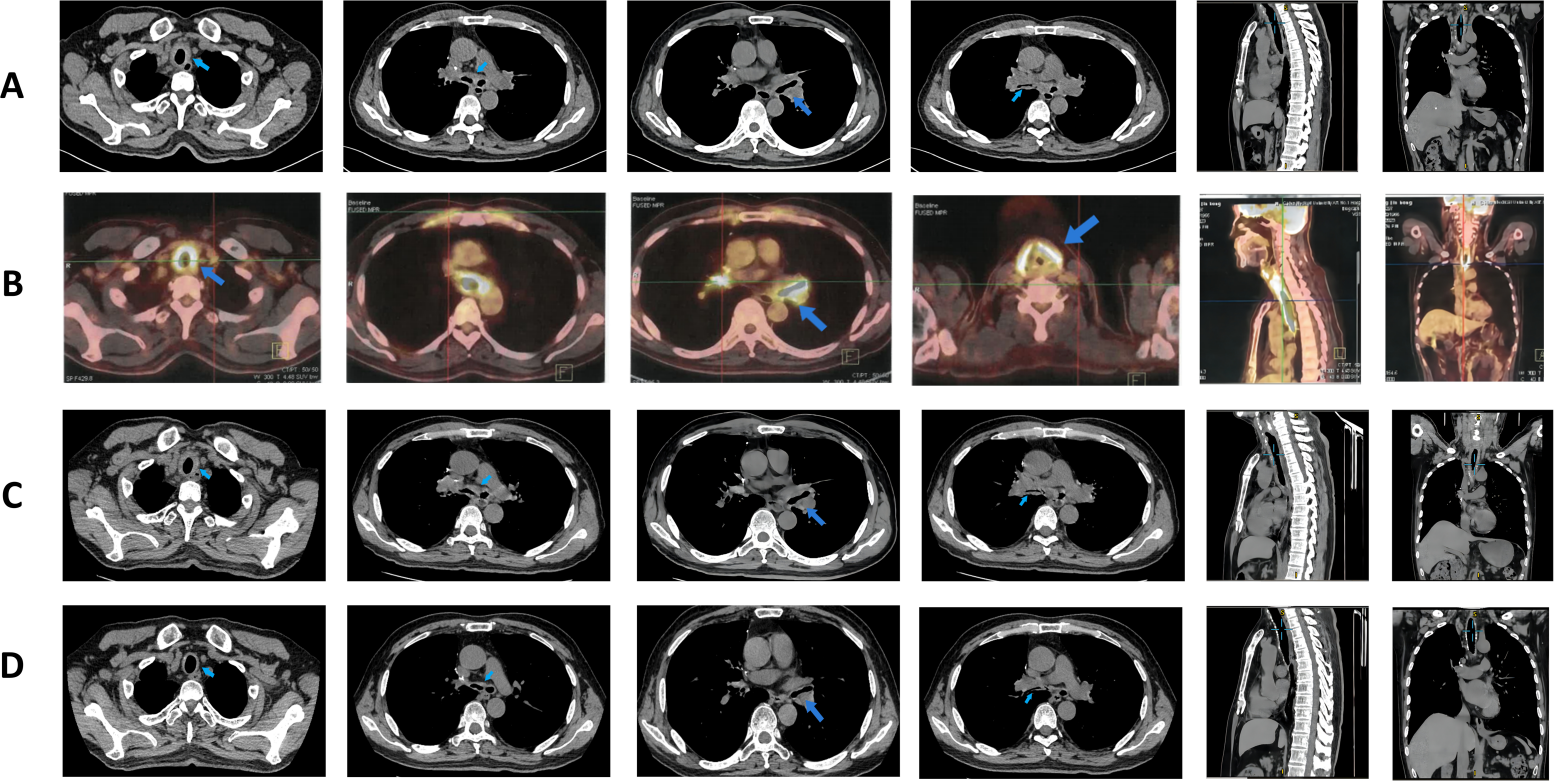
Pulmonary imaging manifestations after disease onset. (A) Enhanced CT of the lungs taken on admission day, revealing wall thickening of the trachea and a double main bronchus, along with bronchial stenosis in the middle and lower lobe of the right lung (treatment: piperacillin sodium and tazobactam sodium + levofloxacin). (B)
